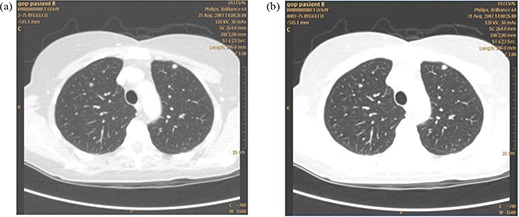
Two low‐dose chest CT images, at the same dose level, at the same position for the same patient: (a) standard reconstruction was used; (b) SharpView postprocessing filter was used.
